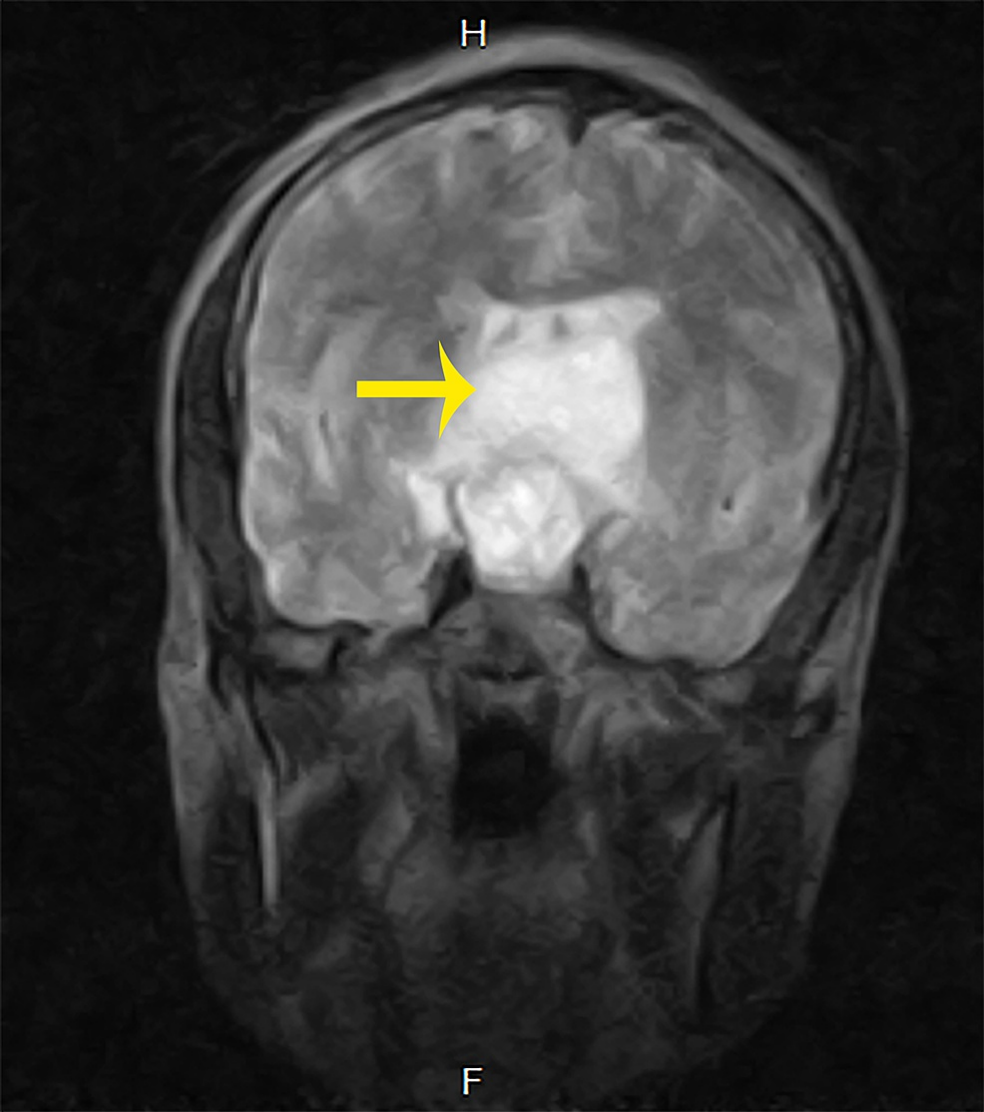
T2-weighted brain MRI showing a suprasellar mass.
